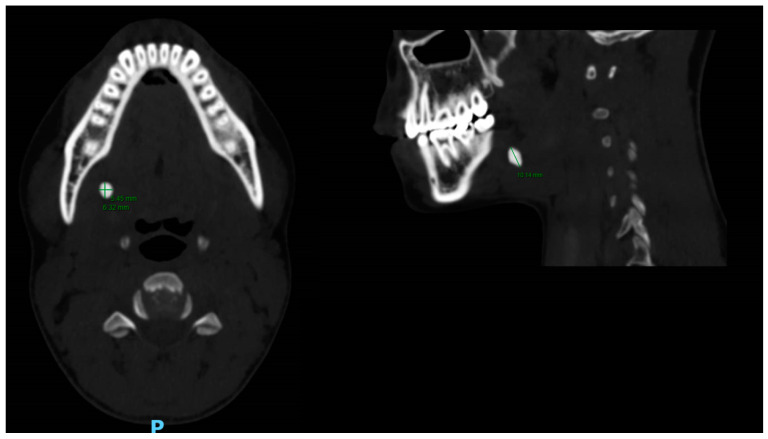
Computed Tomography (CT) of a left submandibular gland sialolith, sagittal section (right), 10.14 mm of the maximal superior-inferior dimension, transverse section (left), dimensions: 5.45 mm latero-medial by 6.32 mm anterior-posterior.
